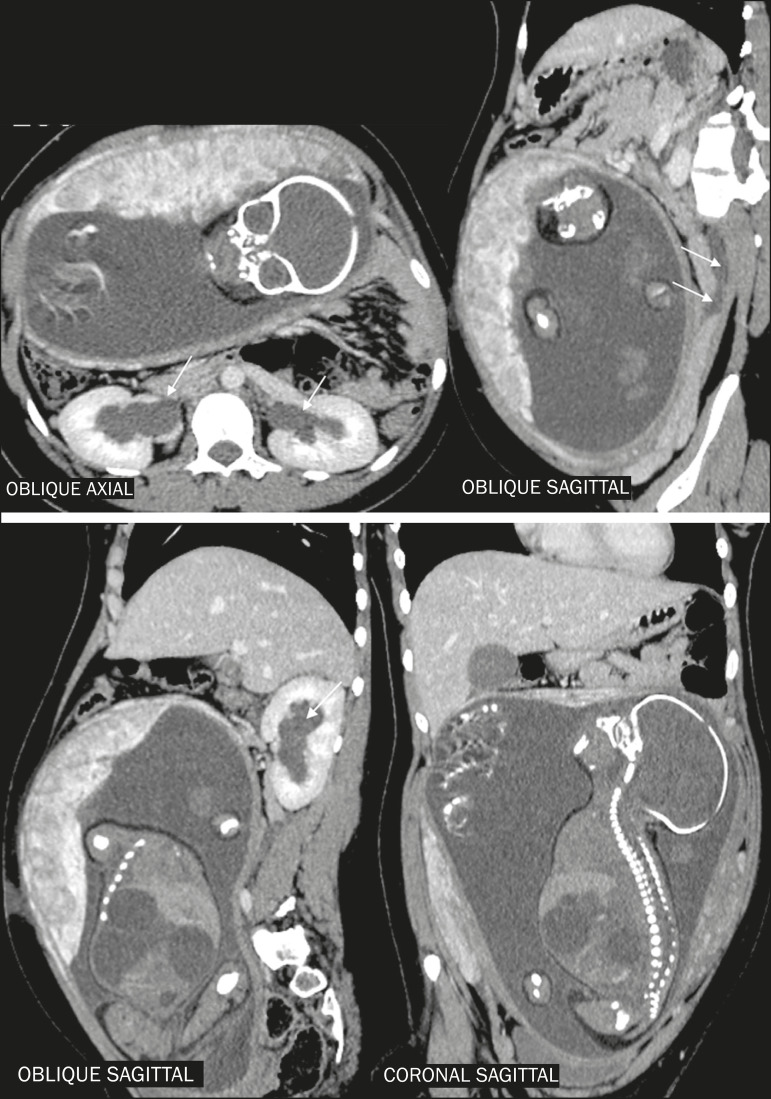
Reconstructions of contrast-enhanced CT scans in the oblique axial, sagittal, and coronal planes showing a patient with physiological hydronephrosis in pregnancy. Note the mild bilateral dilation of the renal pelvis and extrinsic compression of the left ureter by the gravid uterus (arrows). Another incidental finding was dilation of the bowel loops of the fetus, together with polyhydramnios, indicative of intestinal obstruction.
